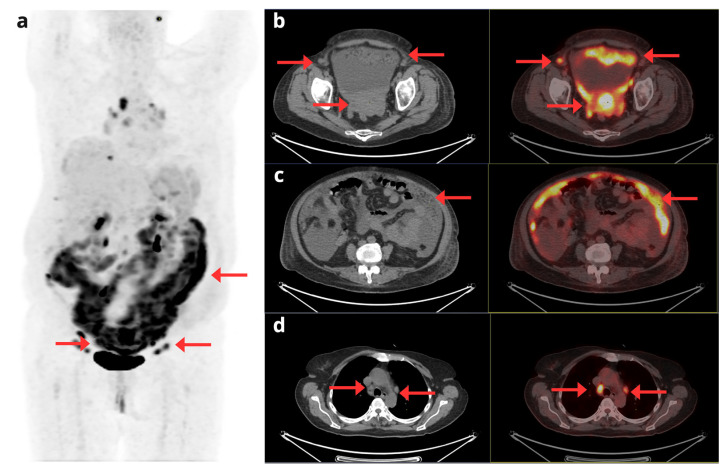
A 70-year-old woman with a diagnosis of stage IV serous EC. The maximum intensity projection PET image (a) shows extensive disease with uterine involvement, supra- and infradiaphragmatic adenopathy, and peritoneal involvement (red arrows). Fused axial CT and [^18^F]F-FDG PET/CT slices (b–d) show foci of pathologic hypermetabolism in the uterus, pelvic lymph nodes, and peritoneum (b); peritoneal implants in the greater omentum (c); and mediastinal adenopathy in the right paratracheal region and the aortopulmonary window (d).
